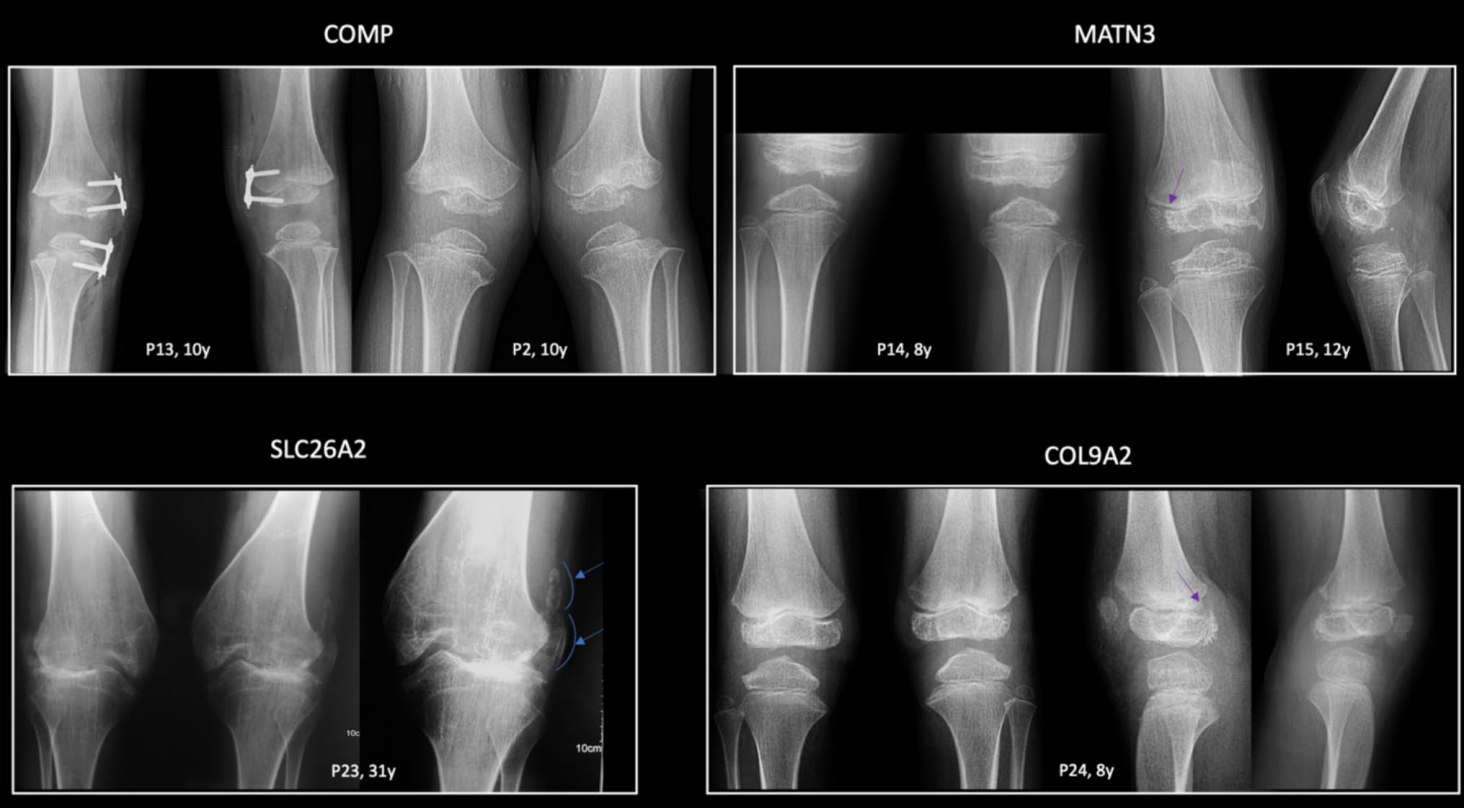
Knee radiographs of the patients. All knee epiphyses are small in patients with *COMP* and *MATN3* variants and irregularly shaped in P2, P14, and P15. P2 and P15 exhibit epiphyses with thin edges at the lateral borders. Additionally, flatness of the femoral condyles, with a shallow intercondylar notch, is observed in P14, P15, and P23. The typical finding of *SLC26A2*-related MED, bipartite patella, is seen in P23 and is indicated with blue lines and arrows. The glacier sign is present in P15 and P24, marked with a purple arrow. P, patient; y, years
